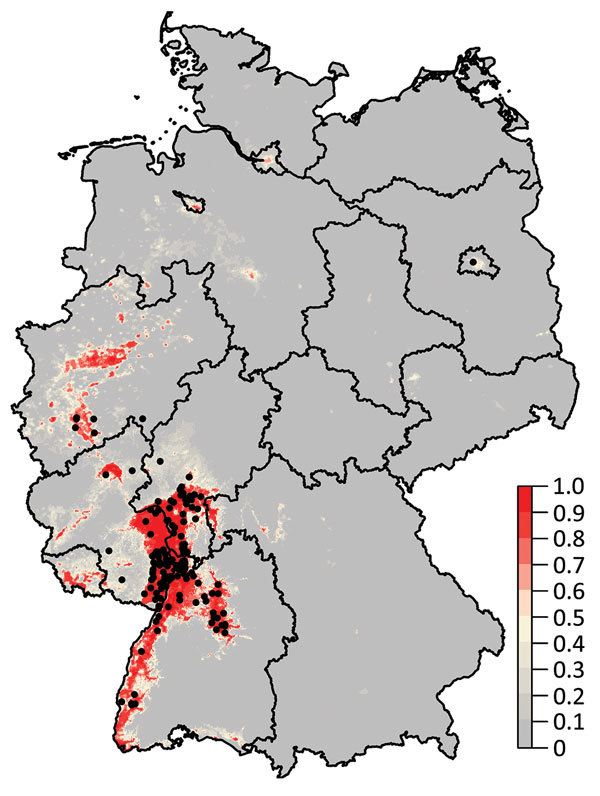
Probability of Usutu virus (USUV) occurrence in Germany derived from 300 boosted regression tree models. Black dots denote sites with dead birds that tested positive for USUV. The color intensity indicates the probability of occurrence of USUV.
